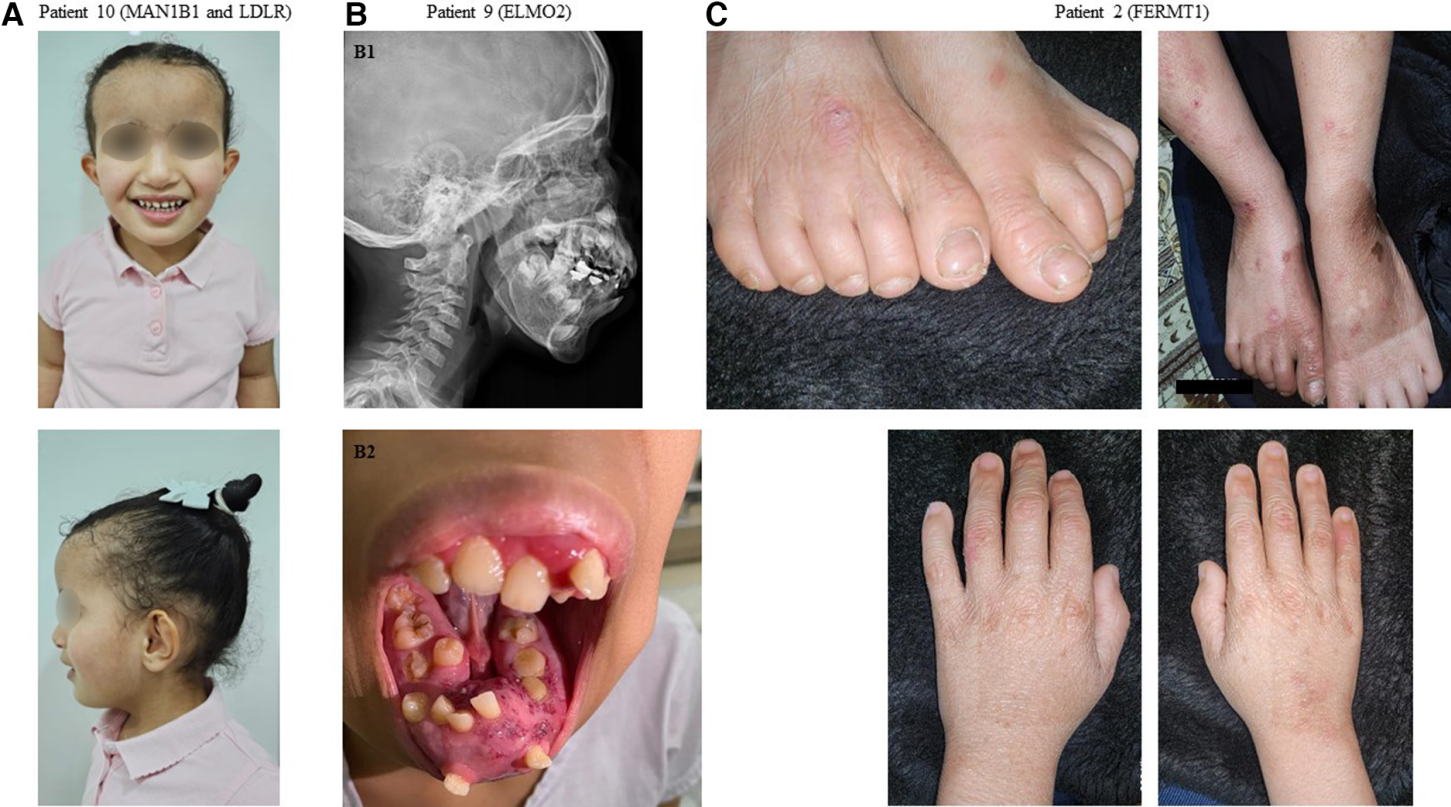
Representative clinical findings in patients with positive molecular diagnosis in our cohort. (A) Patient 10: Front and profile images demonstrating dysmorphic facial features and xanthomas over the antecubital fossa in a patient with a dual diagnosis of Rafiq syndrome (*MAN1B1* variant) and familial hypercholesterolemia (*LDLR* variant). (B1) Patient 9: A radiograph demonstrating mandibular hyperostosis in a patient with primary intraosseous vascular malformation (*ELMO2* variant). (B2) Patient 9: A clinical image demonstrating mandibular hyperostosis. (C) Patient 2: Images of the hands and feet demonstrating skin blistering and fragility in a patient with Kindler syndrome (*FERMT1* variant).
